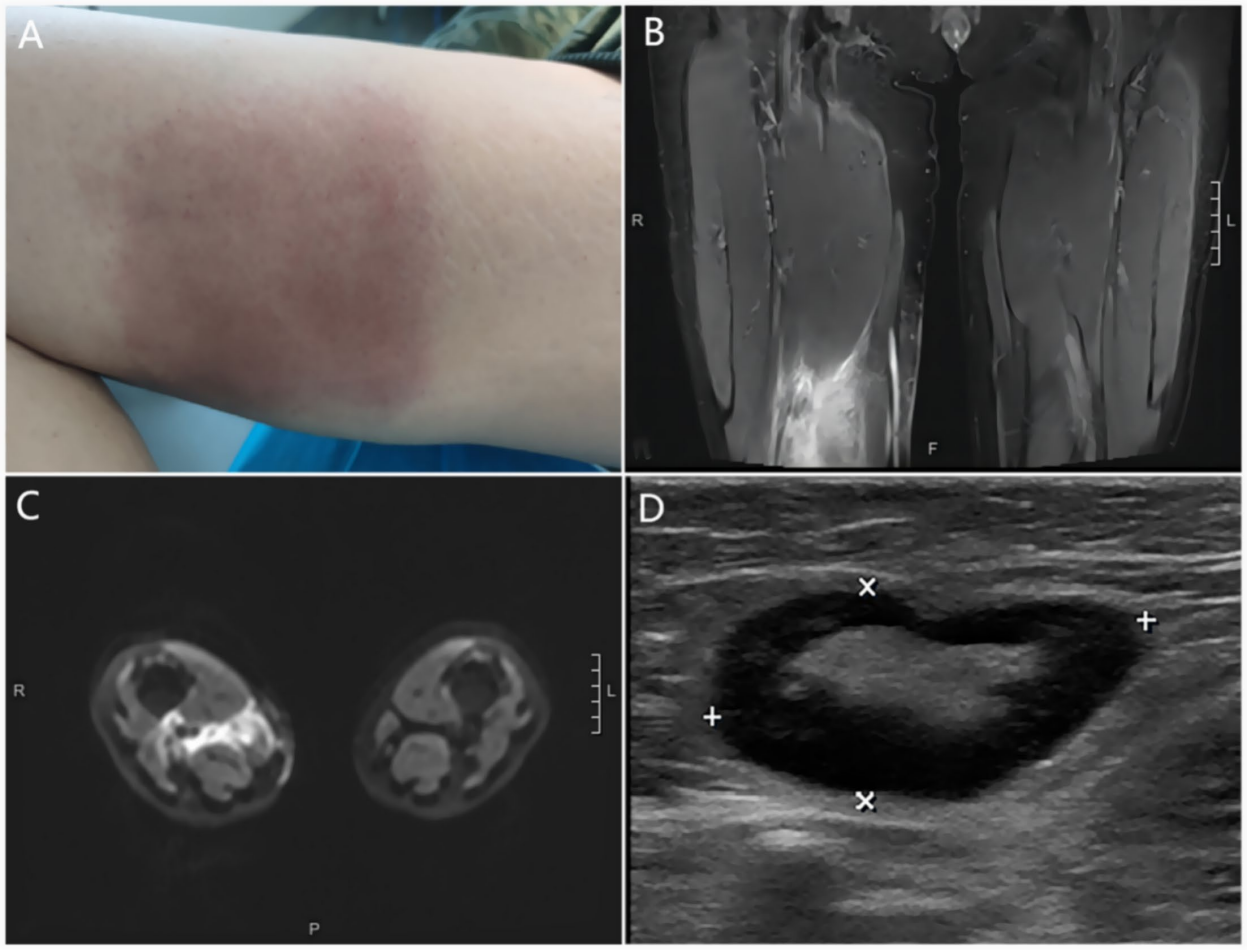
(A) 15 × 15 cm square-shaped edematous, infiltrative, well-demarcated erythema on the right medial femur with a tough texture. (B) Coronal MRI of the lower limbs showing abnormal signals in the right lower posterior femoral muscle group and muscle spaces. (C) Transverse axial MRI of the lower limbs showing abnormal signals in the right lower posterior femoral muscle group and muscle spaces. (D) Ultrasonography of the right groin showing an enlarged lymph node with a clear border (2.7 × 1.3 cm).
